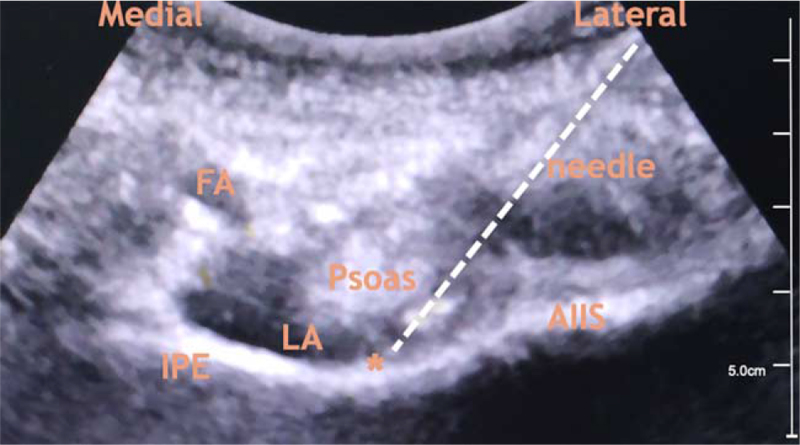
Ultrasonographic view during PENG block. AIIS = anterior inferior iliac spine, FA = femoral artery, IPE = iliopubic eminence, LA = local anesthetic, PENG = pericapsular nerve group block. Asterisk (_∗_): target for local anesthetic injection.
